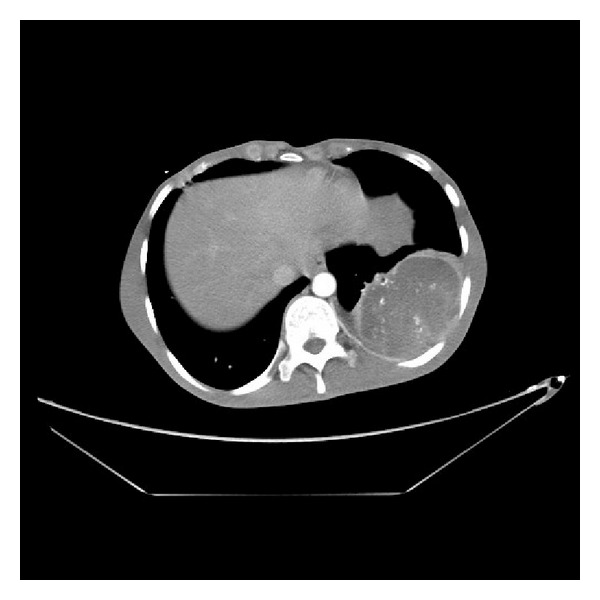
Abdominal computed tomography scan revealed renal metastases of osteosarcoma.
